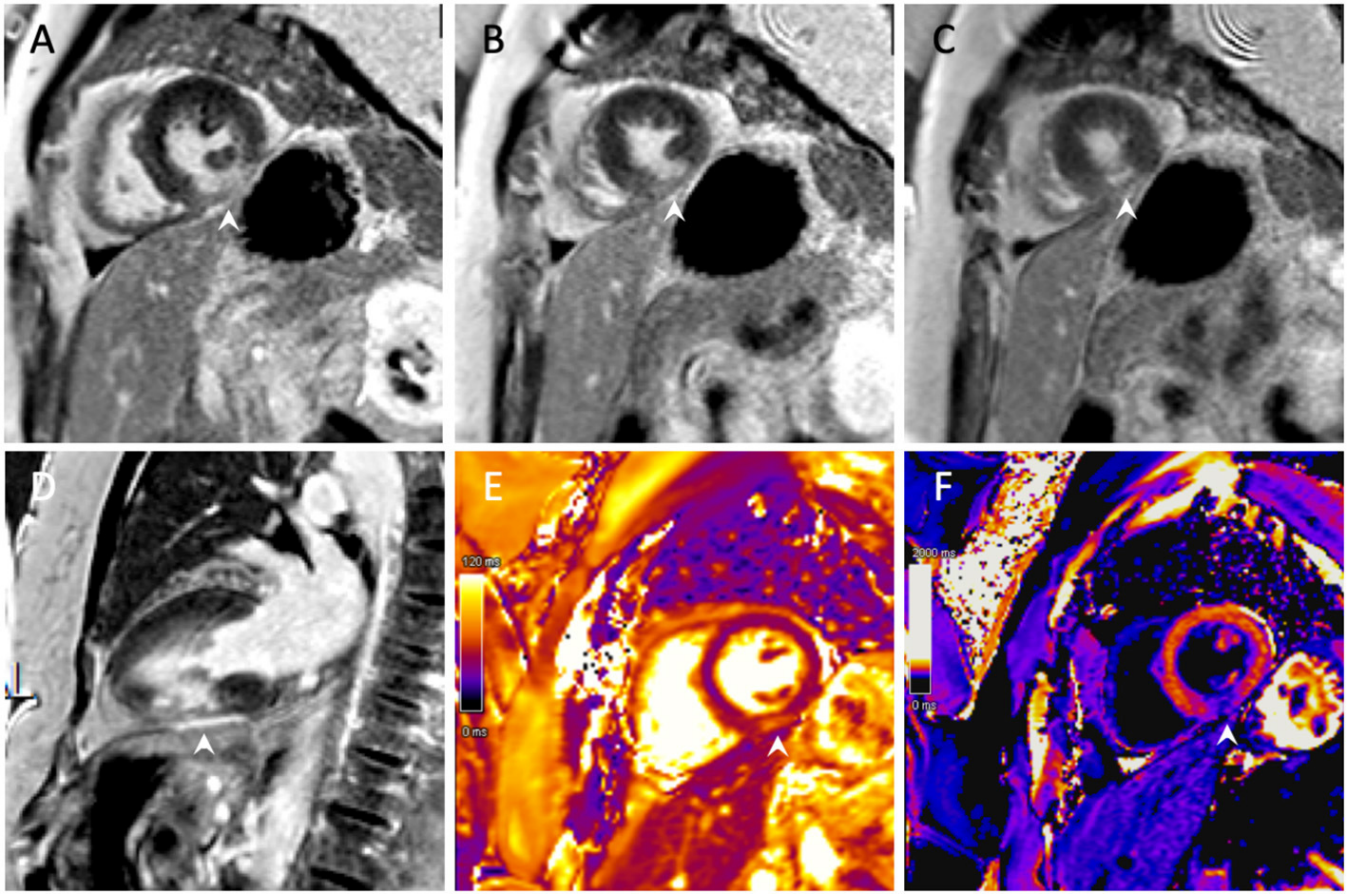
Cardiac magnetic resonance imaging with gadolinium. (A–C) Short-axis view with transmural late gadolinium enhancement of the mid-inferior left ventricular wall with subendocardial extension toward the apex. (D) Two-chamber view demonstrating an inferior infarction of the mid segment with extension toward the apex. (E) T2 imaging with oedema of the mid-inferior segment. (F) Post-contrast T1 mapping of the infarct.
